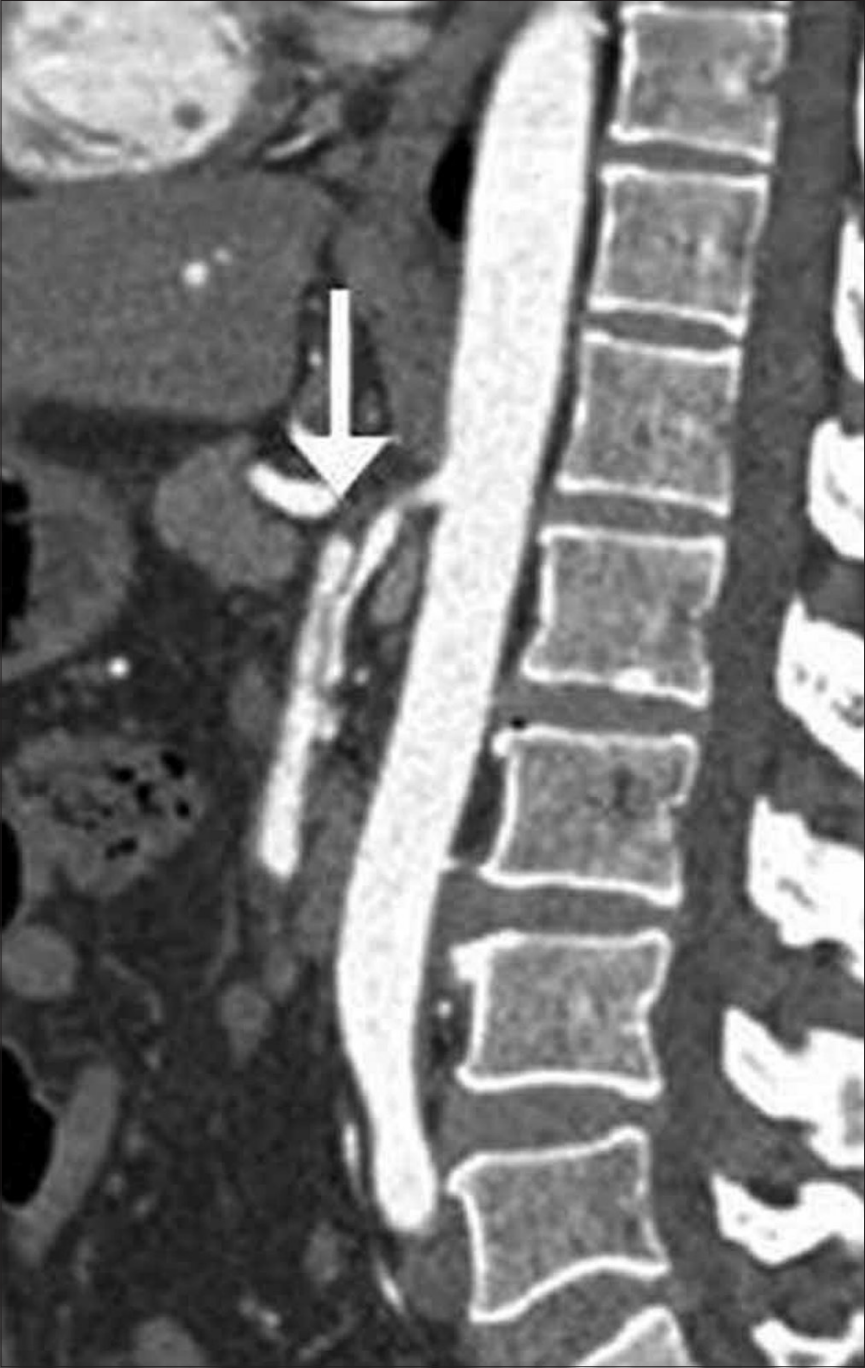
Sagittal, contrast-enhanced, arterial phase, maximum intensity projection CT scan shows a dissection flap (arrow) in the superior mesenteric artery
